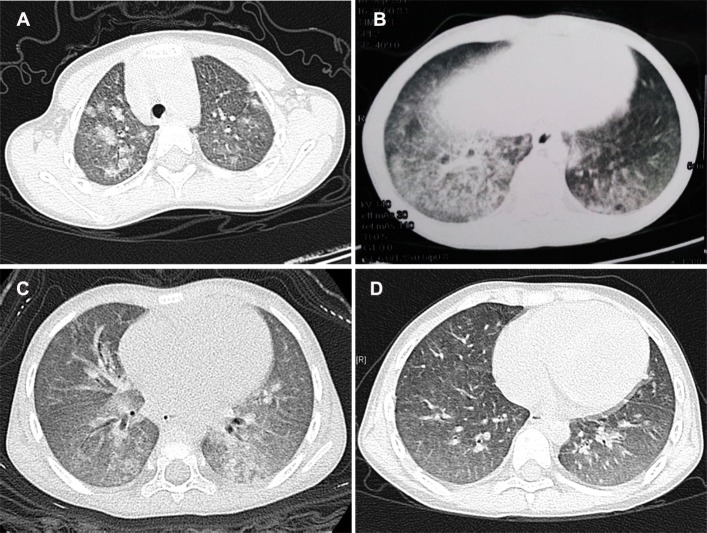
Lung CT of MPA patients with diffuse alveolar hemorrhage. (A): Shadows of gobbets (arrow) were observed in both lungs, and especially in the right one (patient 2). (B) and (C): Chest CT of patients 3 and 7, with ground glass opacity observed in the imaging. (D): Lung CT of patient 3 after 2 months of therapy, with the ground glass opacity having disappeared in contrast to 1B.
